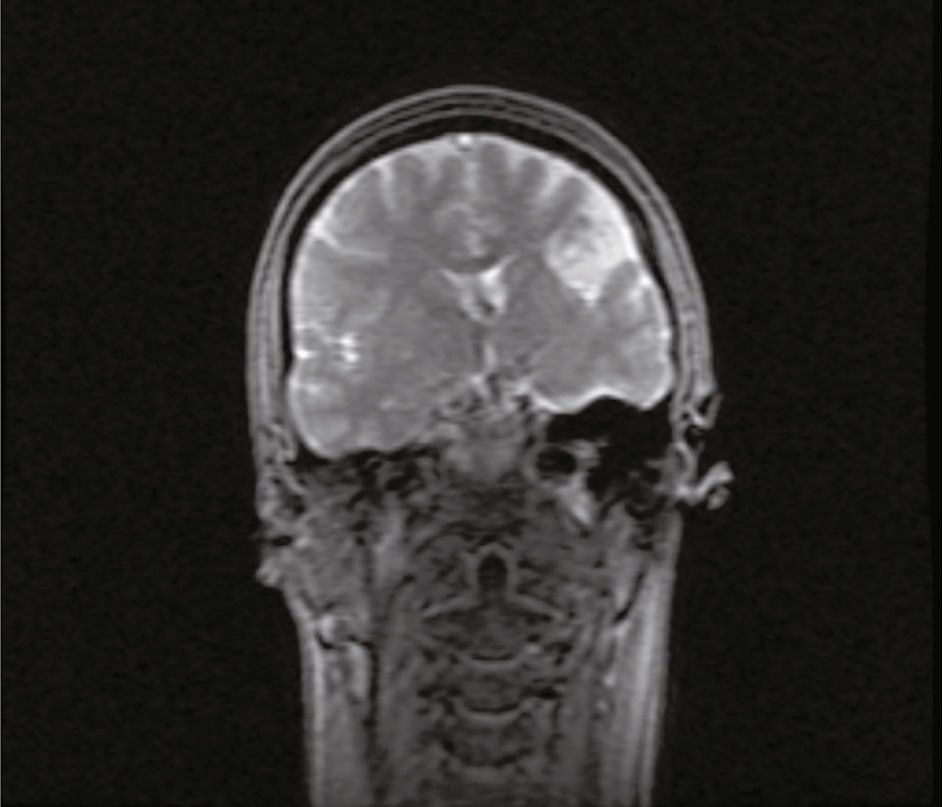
Coronal T2-weighted view showing the fronto-opercular porencephalic cyst with CSF inside.
